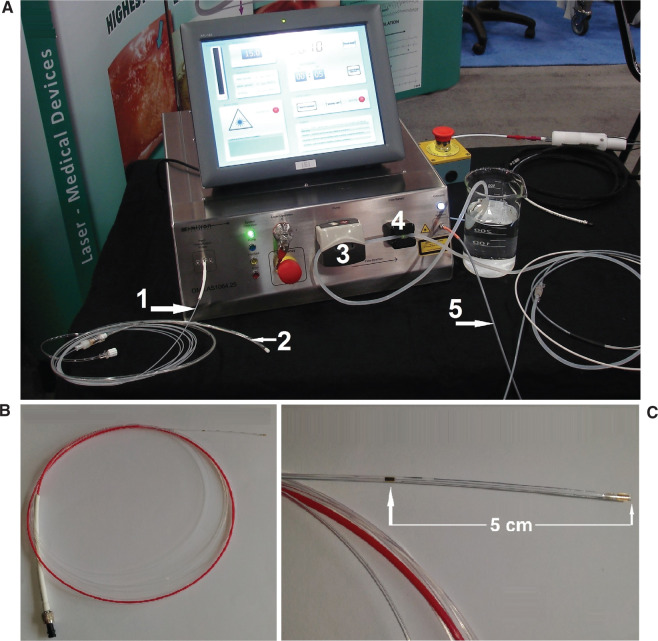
A: Frontal view of the custom-made, 1064-nm MediLas D diode laser (LasCor GmbH, Taufkirchen, Germany) with (1) the optical fiber of the SLLS and (2) its distal end with the light sensor fed into a transparent esophageal probe. Additionally, (3) the roller-pump for saline irrigation of the RLC, (4) ultrasound flow control of catheter irrigation, and (5) the open-irrigated ELMA RLC itself are indicated. B: Overview of the transesophageal SLLS catheter. C: The SLLS catheter’s distal end containing the 5-cm-long light sensor with X-ray markers.
